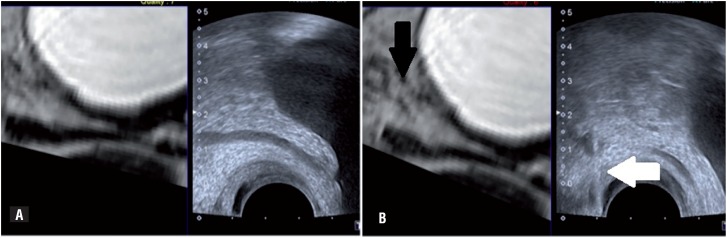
A) Tomography and ultrasound images fusion, showing images synchronization. B) On the left, arrow (black) shows the position of the thickened vas deferens (CT); on the right, the biopy needle into the target lesion (white arrow).
